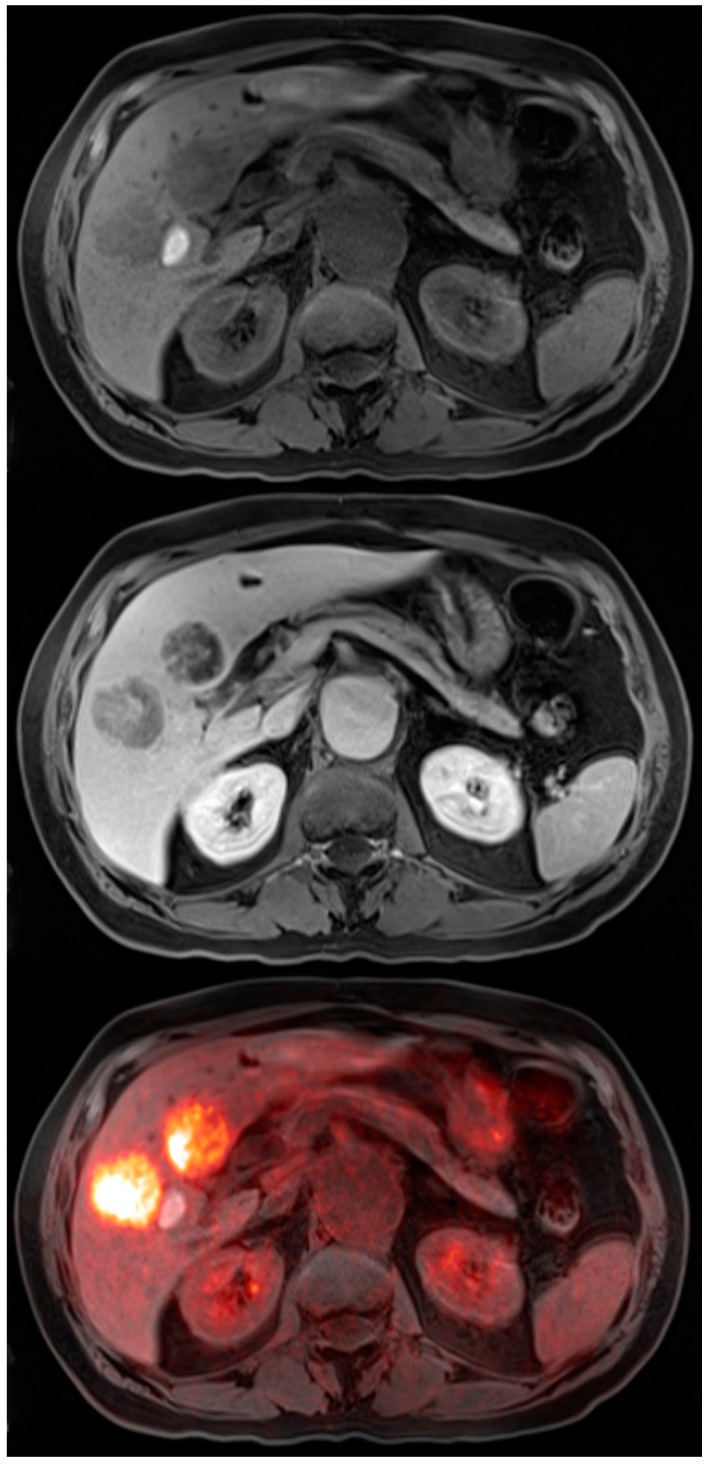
72-year-old male with colon cancer. T1 precontrast transaxial image shows two low signal masses in segment 4B and 5 of the liver (*top*). T1 post contrast transaxial imaging with gadoxetate disodium reveals heterogenous enhancement of these two masses consistent with metastatic colon cancer (*middle*). PET-MRI transaxial fusion image confirms malignancy by demonstrating intense FDG activity (*bottom*). Also note the adjacent benign hemorrhagic cyst with high T1 signal that does not exhibit increased metabolic activity on the fusion images.
